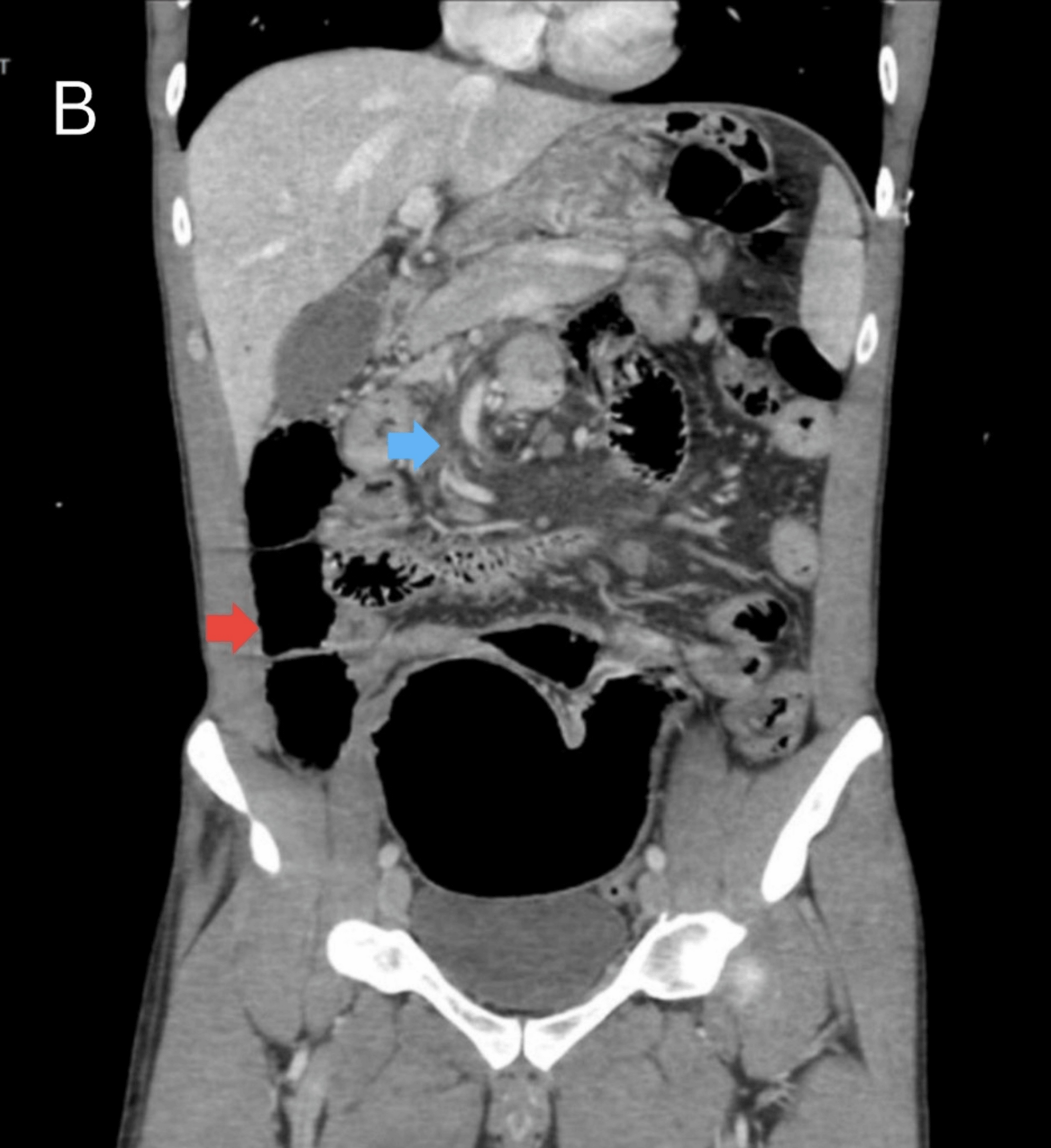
Coronal CT of the abdomen The coronal image of the abdomen further demonstrates diffuse bowel loop distention (red arrow). The configuration of distended bowel loops along with mesenteric crowding and twisting with displacement of vasculature (blue arrow) raises suspicion for a closed-loop obstruction with mesenteric volvulus. CT: computed tomography
